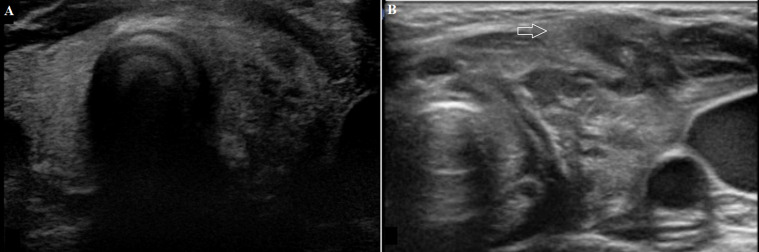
second sonographic evaluation: A) the inflammatory lesion extends between the airway and left carotid artery, centrally the sinus track is obvious; B) axial view of thyroid gland, abnormal enlargement and heterogeneity of left thyroid lobe, compared with normal right thyroid lobe
